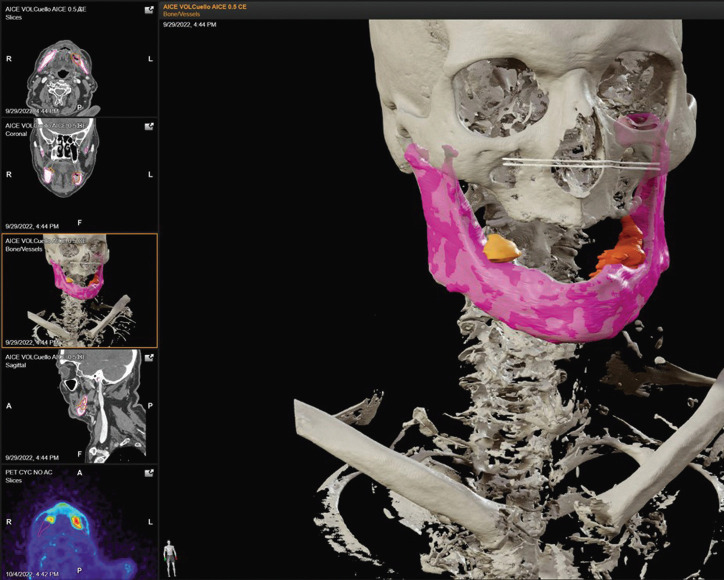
Superimposition of PET and CT in Brainlab. Segmentation of the mandible and the two le-sions to treat. In this case, the lesion located in the left mandibular body (orange) will be guided.
